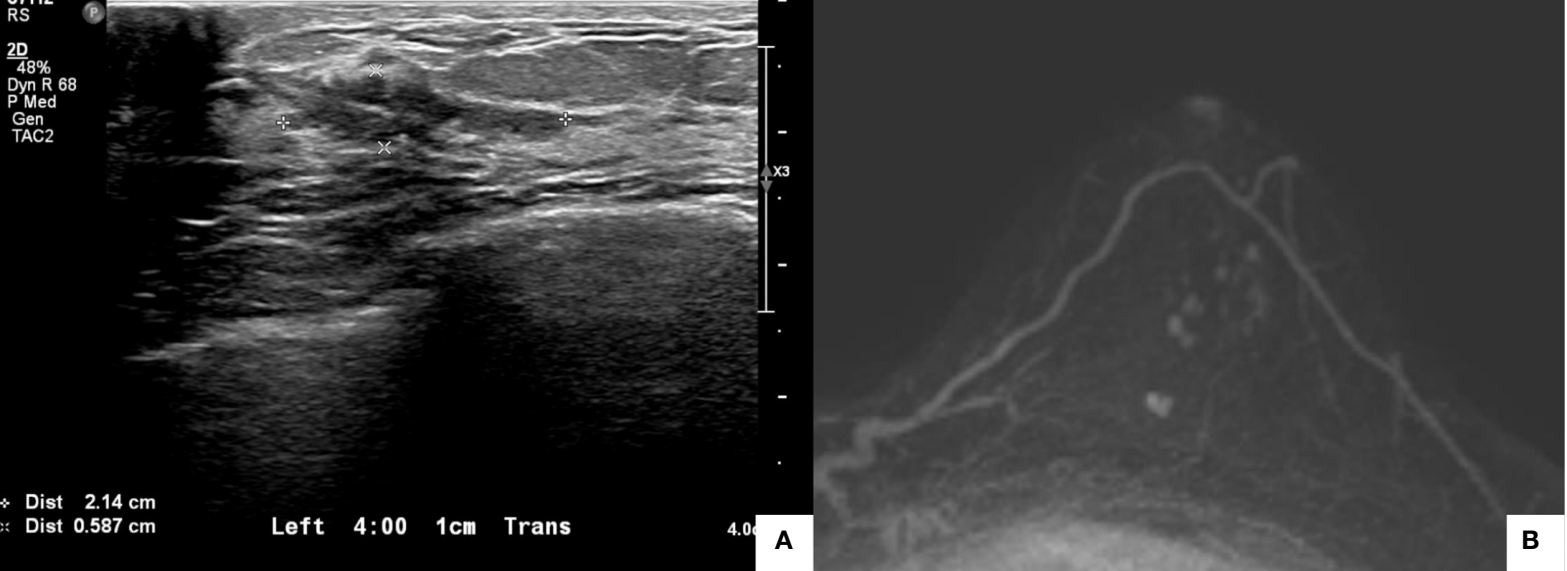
Posttreatment imaging evaluation. Ultrasonography showed that the dimensions of the malignant mass had decreased to 2.1 × 1.0 cm (A). Magnetic resonance imaging showed that the biopsy-confirmed malignant mass and multiple suspicious daughter nodules (total extent measuring 2.1 cm) in the left breast had decreased in size (B).
